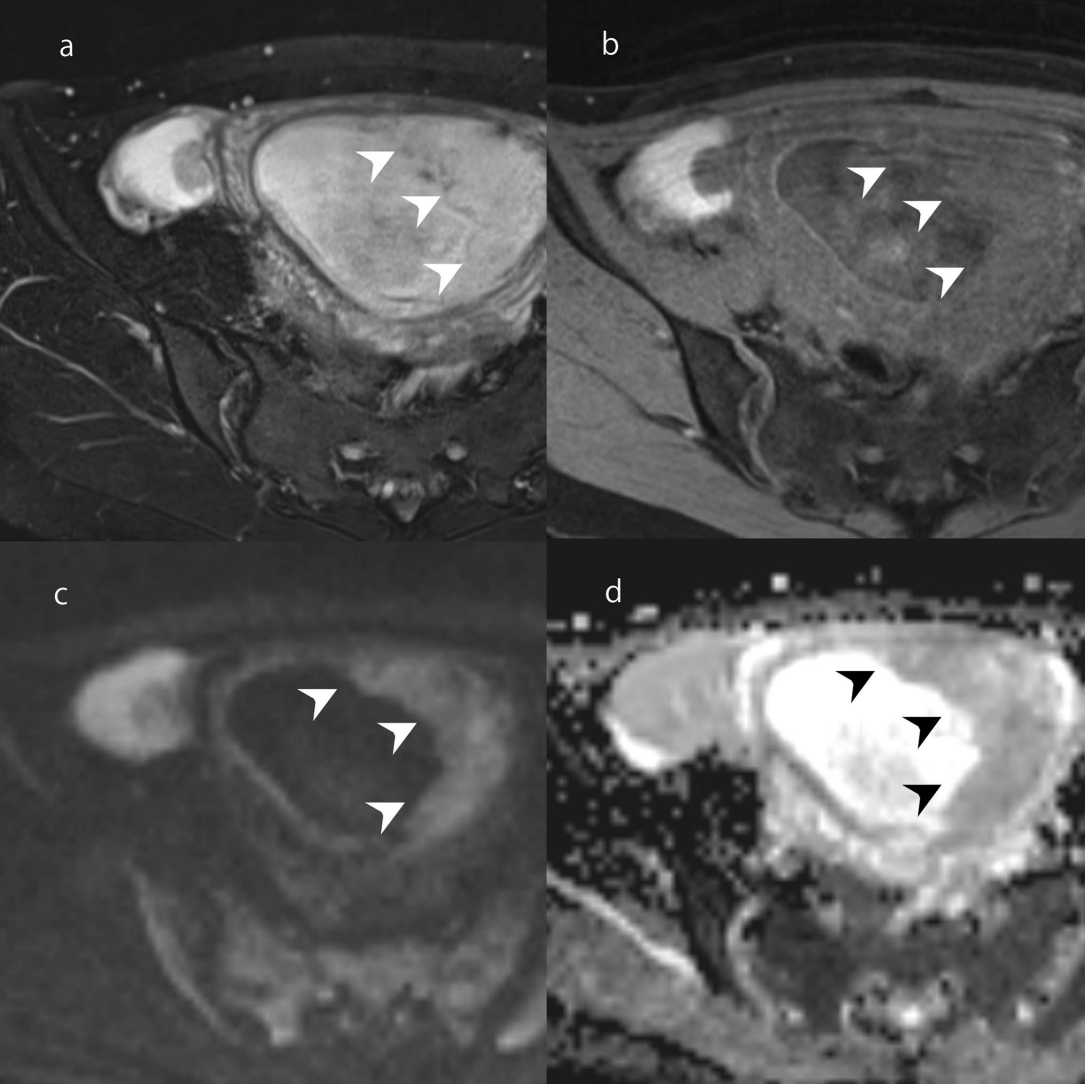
Decidualized endometriosis in a woman in her 20s at 14 weeks of pregnancy. a A cystic mass with a mural nodule is observable in the right ovary. On FS-T2WI, the mural nodule is as hyperintense as the placenta (arrowhead). b On FS-T1WI, the mural nodule is iso-intense compared with the placenta (arrowhead). c On DWI, the mural nodule is as hyperintense as the placenta (arrowhead). d On ADC maps, the mural nodule is as hypointense as the placenta (arrowhead)
